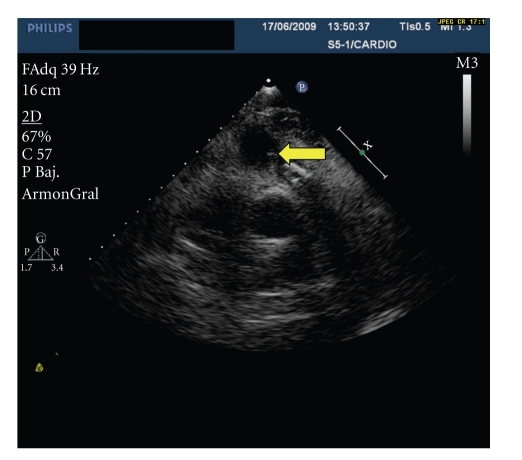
Transthoracic echocardiography parasternal section showing a thickened and calcified pulmonary valve with adhered vegetations.
